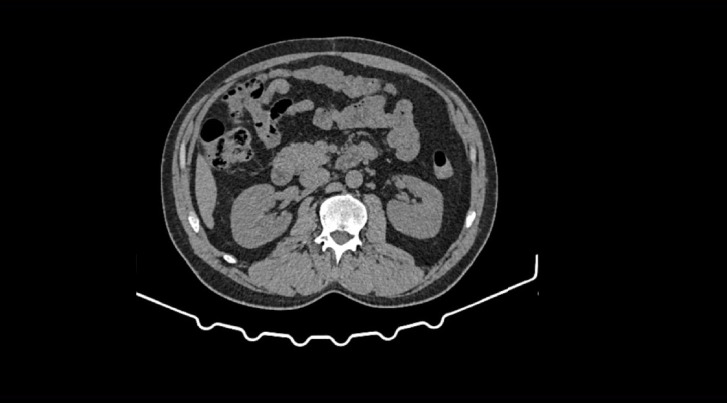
CT scan 8 months after surgery for the retroperitoneal cyst shows normal positioning of the right kidney and no evidence of recurrence.
